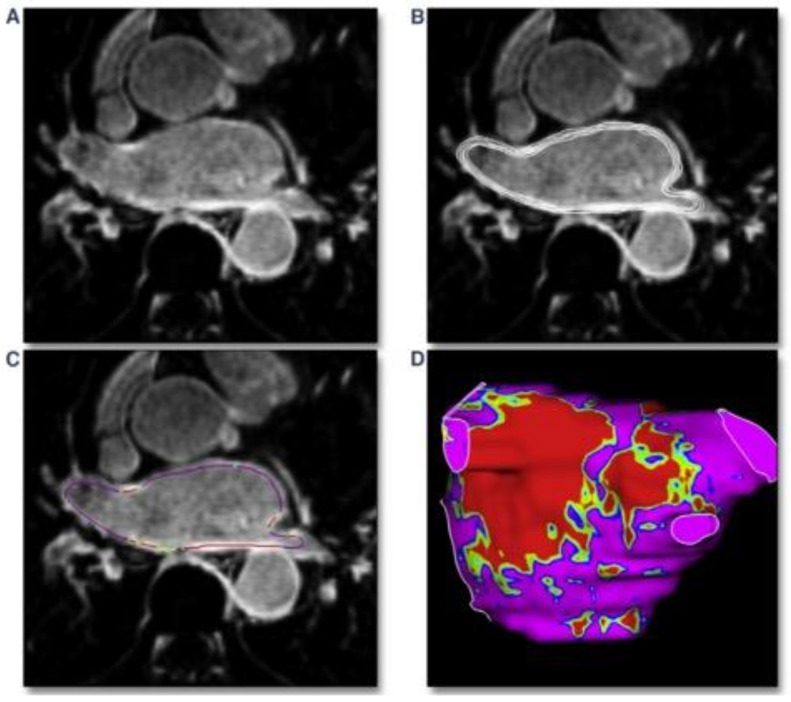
Post-processing phase of left atrium detected with cardiac MRI basing on pixel signal intensity. (A): MRI-LGE scan of the left atrium. (B): manual segmentation of the atrial wall with analysis of multiple layers from endocardium to epicardium. (C): pixel intensity map creation along the atrial wall. (D): 3-dimensional shell of the left atrium with pixel intensity map based on different colors; red: dense scar, green/yellow: border zone, purple: healthy tissue. Requested permission from [49].
